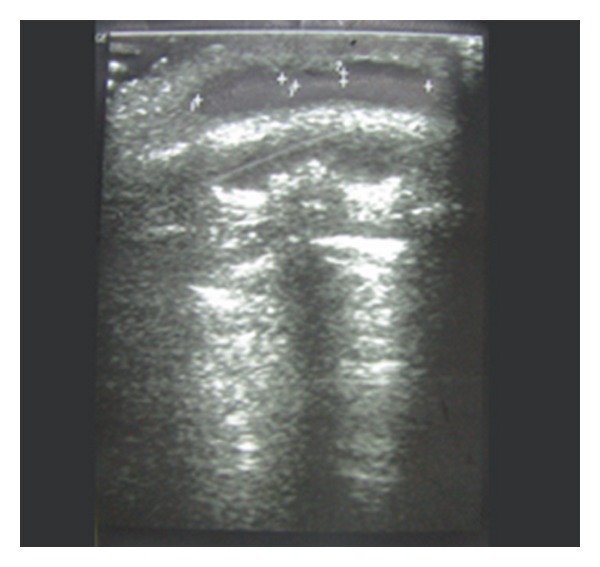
Sonogram of patient shows hypoecho subcutaneous mass.
